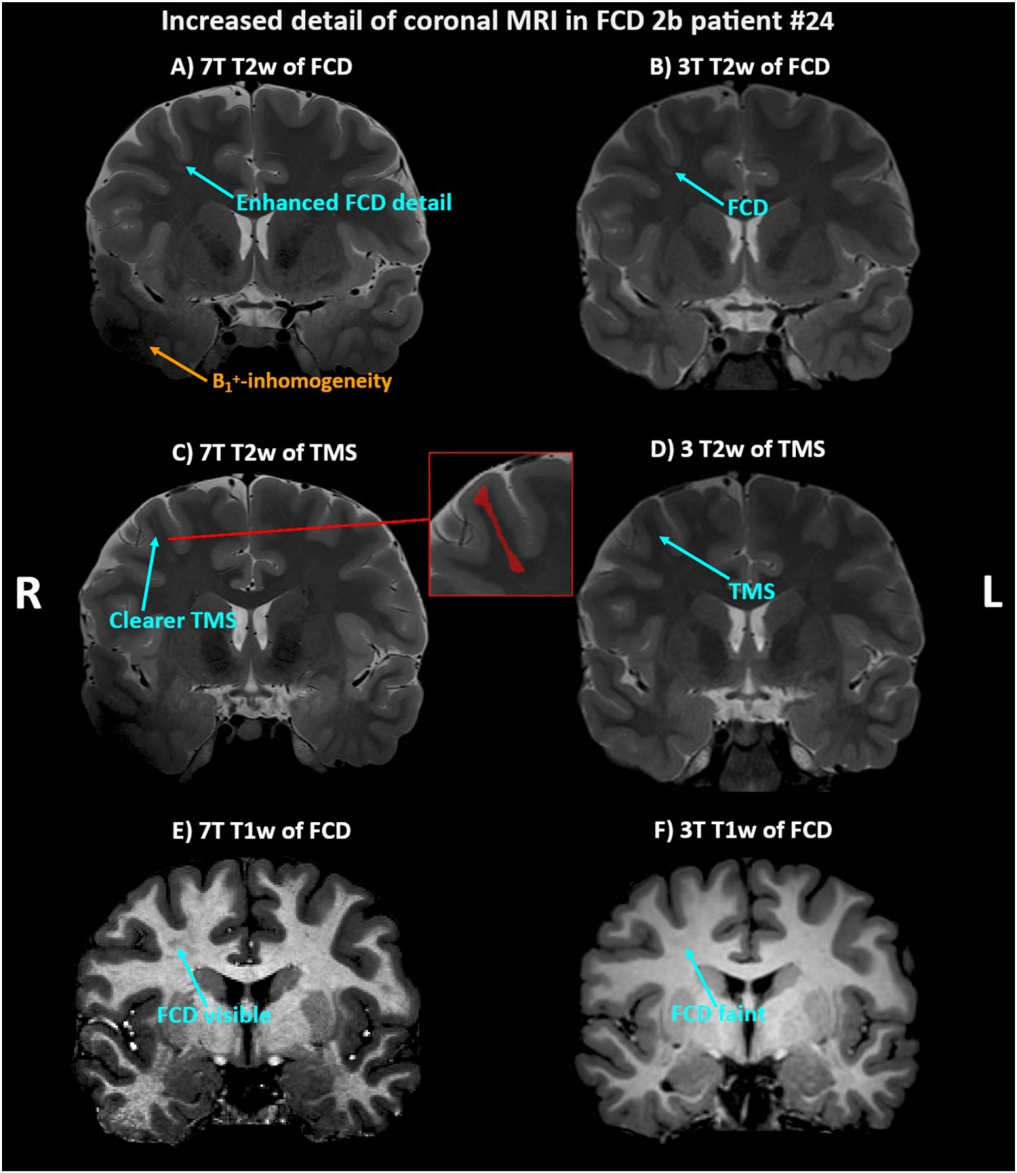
In patients such as #24, the 7 T epilepsy consensus protocol adds visible details that could be useful for neurosurgical planning, such as the enhanced delineation of an focal cortical dysplasia (FCD) (A) vs. B)) or the accompanying trans-mantle sign (TMS) in image (C) vs. D)) of this example. On T1w imaging (E vs F), the FCD is clearly visible at 7 T, while inconspicuous at 3 T. Arrows highlight regions of interest for pathology or artifacts. *T1w*  T1-weighted, *T2w*  T2-weighted, *7 T* 7 Tesla, *3 T* 3 Tesla, *B1*^+^  radio frequency excitation, *R* right side, *L* left side
